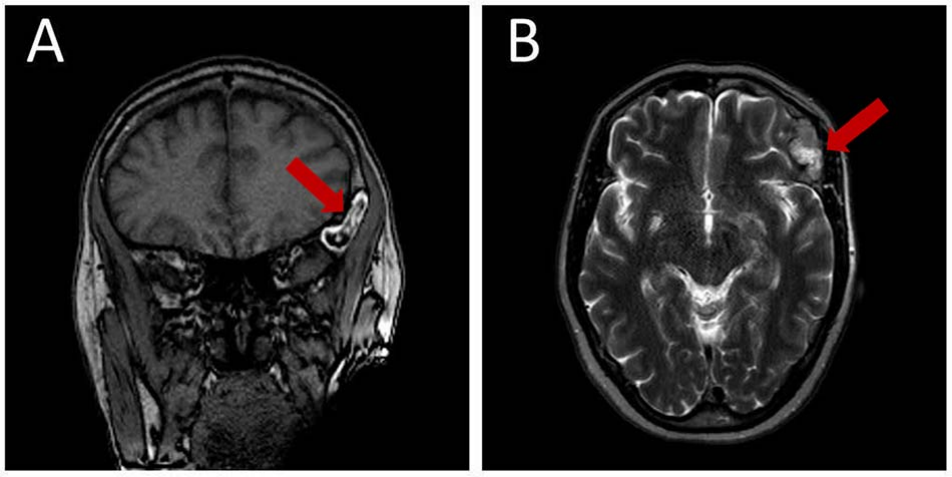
Pre-operative brain MRI exhibiting: (A) a high-intensity lesion in the left fronto-zygomatic region on coronal T1-weighted images (T1WI), and (B) Axial T2-weighted images (T2WI) depicting variable signal intensity ranging from iso- to hyperintense.
